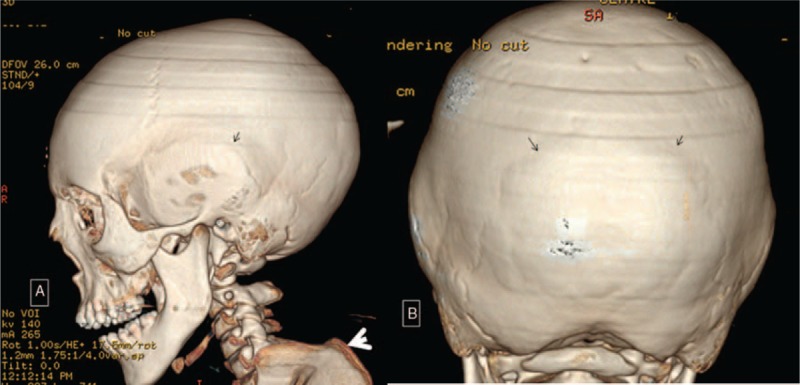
(A) 3D-CT scan of the mother skull showed bilateral bulging of the temporals. Synostosis of the sagittal suture with posterior bulging deformity akin to her son. Note the bulging of the synostosed squamosal suture (arrow) and the massive kyphosis (arrow head). Coronals are normal. (B) Mother 3D reconstruction CT scan showed diffuse synostosis of the lambdoids (arrows). Note the marked bossing of the occipital area. CT = computed tomography.
